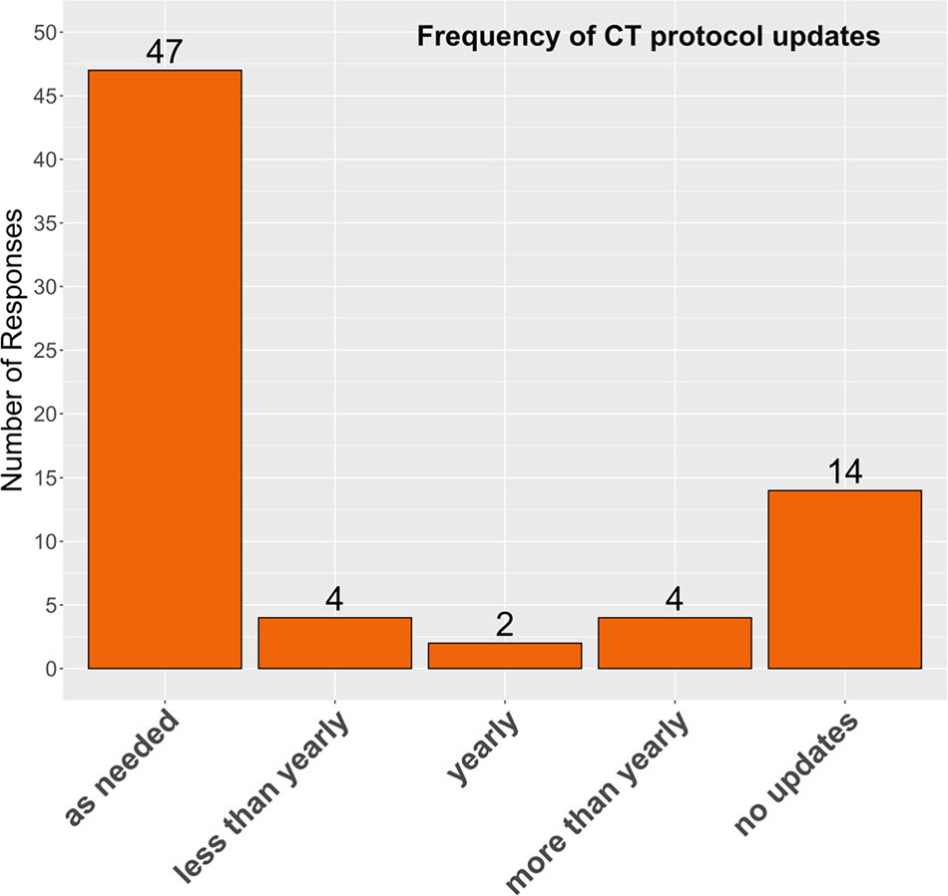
Frequency of CT image acquisition protocol updates per lung cancer screening facility
